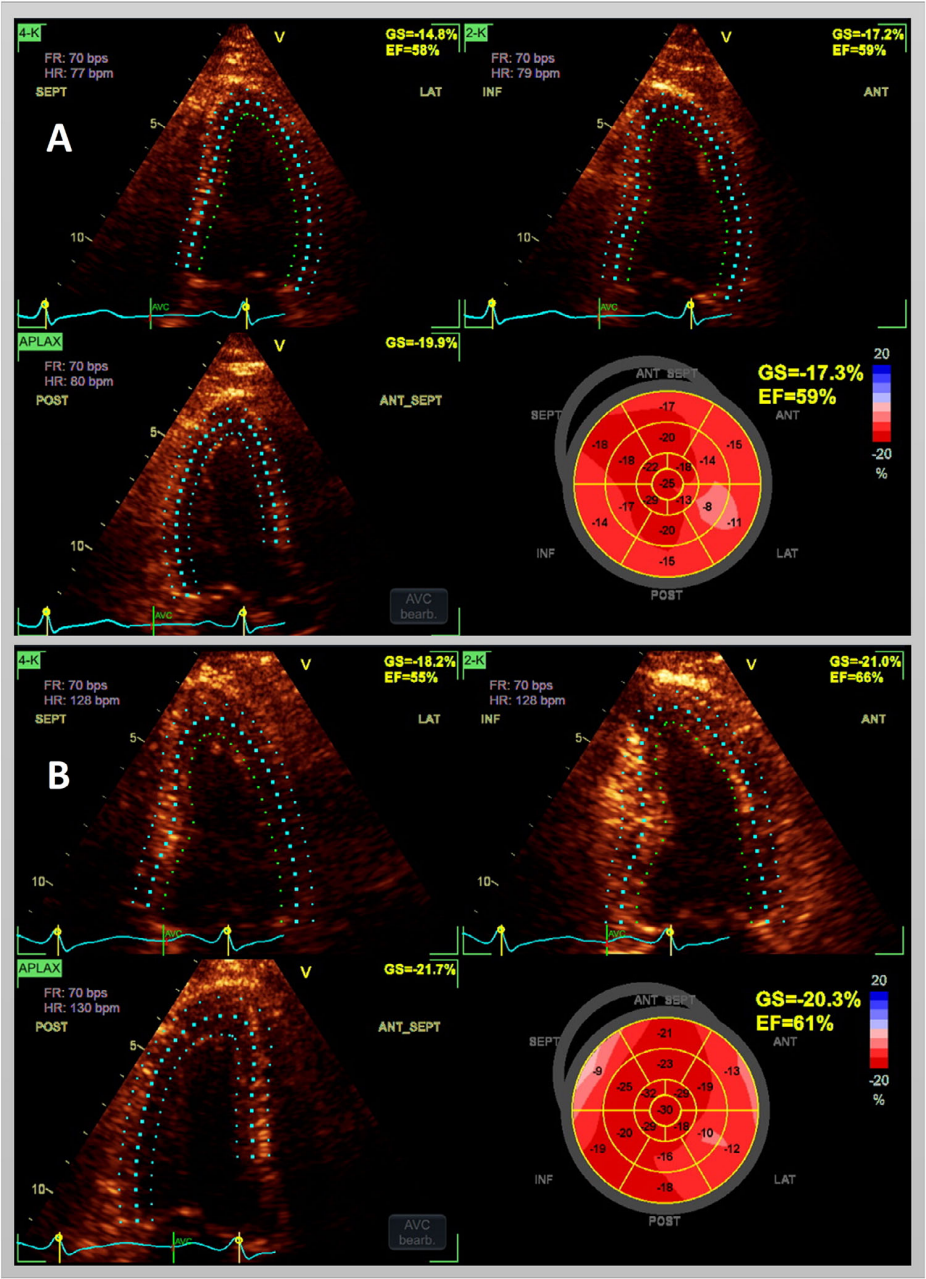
A 69‐year‐old female patient with a history of PCI of the left anterior descending artery following an acute coronary syndrome. The figure demonstrates stress echocardiography performed on a S70N system (GE Vingmed Ultrasound AS, Horten, Norway) with two‐dimensional left ventricular longitudinal strain imaging. At rest (A), there is a reduction in regional longitudinal strain in the anterior wall, which is more pronounced in the anterolateral wall. Under stress, using a semisupine bicycle test at maximum exertion (100 watts) (B), global and regional longitudinal strain improves, indicating good contractile reserve and no new signs of ischemia. PCI, percutaneous coronary intervention.
